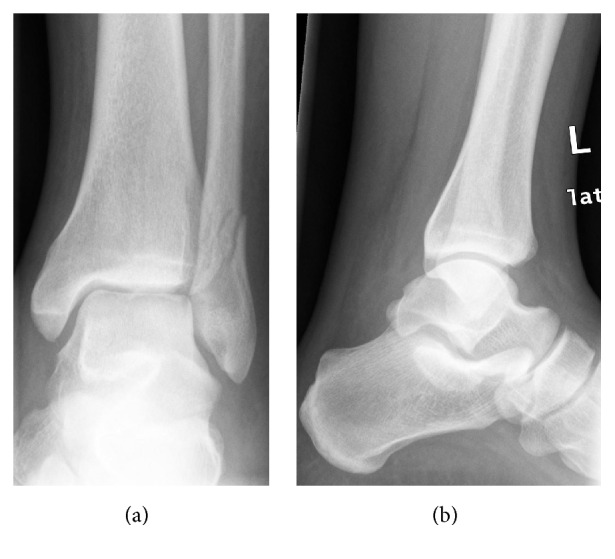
Plain (a) anteroposterior and (b) lateral radiographs of the left ankle on initial presentation revealing a nondisplaced, short, oblique fracture of the lateral malleolus, Danis-Weber B.
